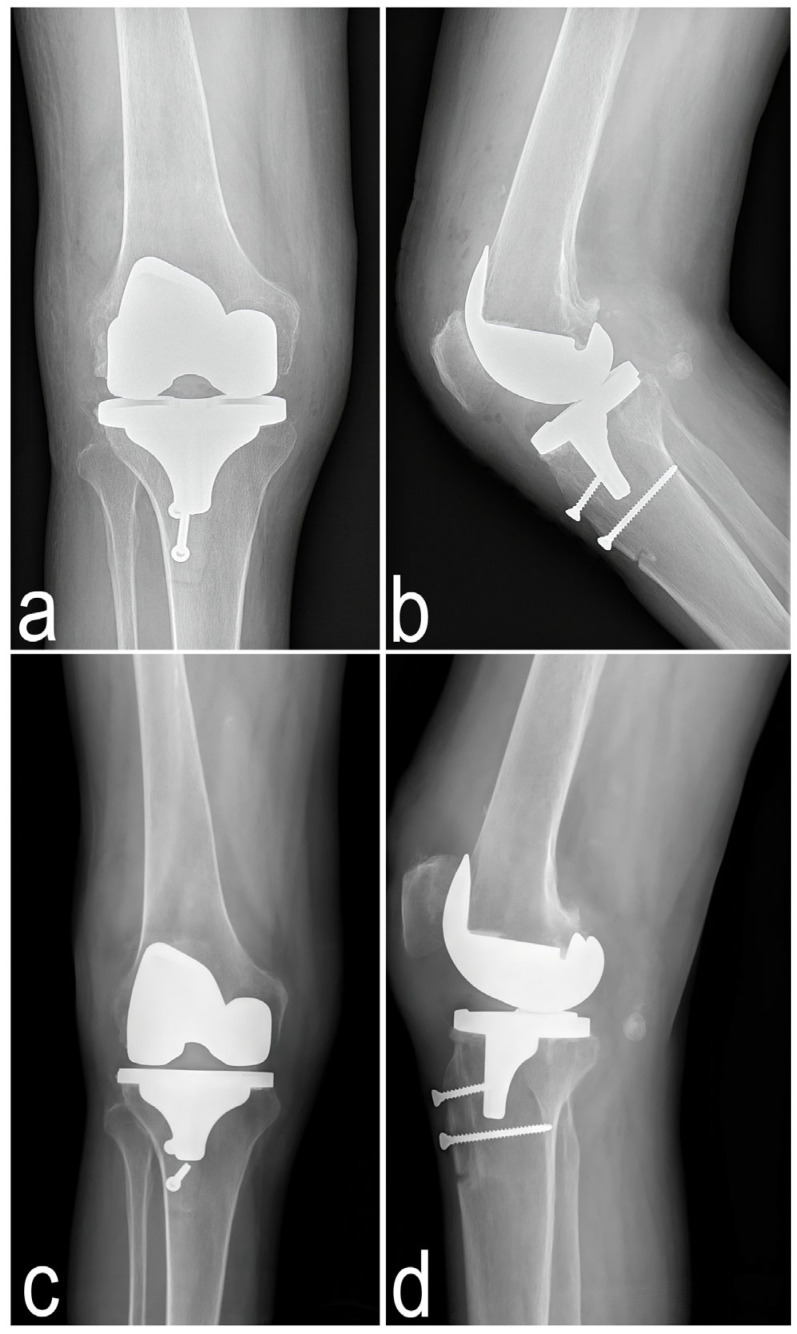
Postoperative X-ray of the right knee. The first postoperative day: (c)—Standing anteroposterior view; (d)—Lateral view. Three months after total knee arthroplasty: (a)—Standing anteroposterior view; (b)—Lateral view.
